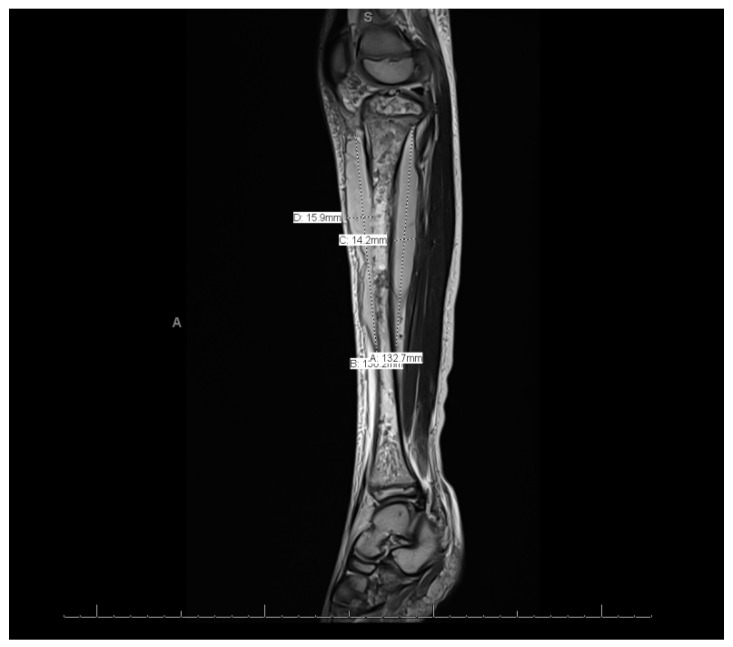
Magnetic resonance imaging of the whole left leg.
